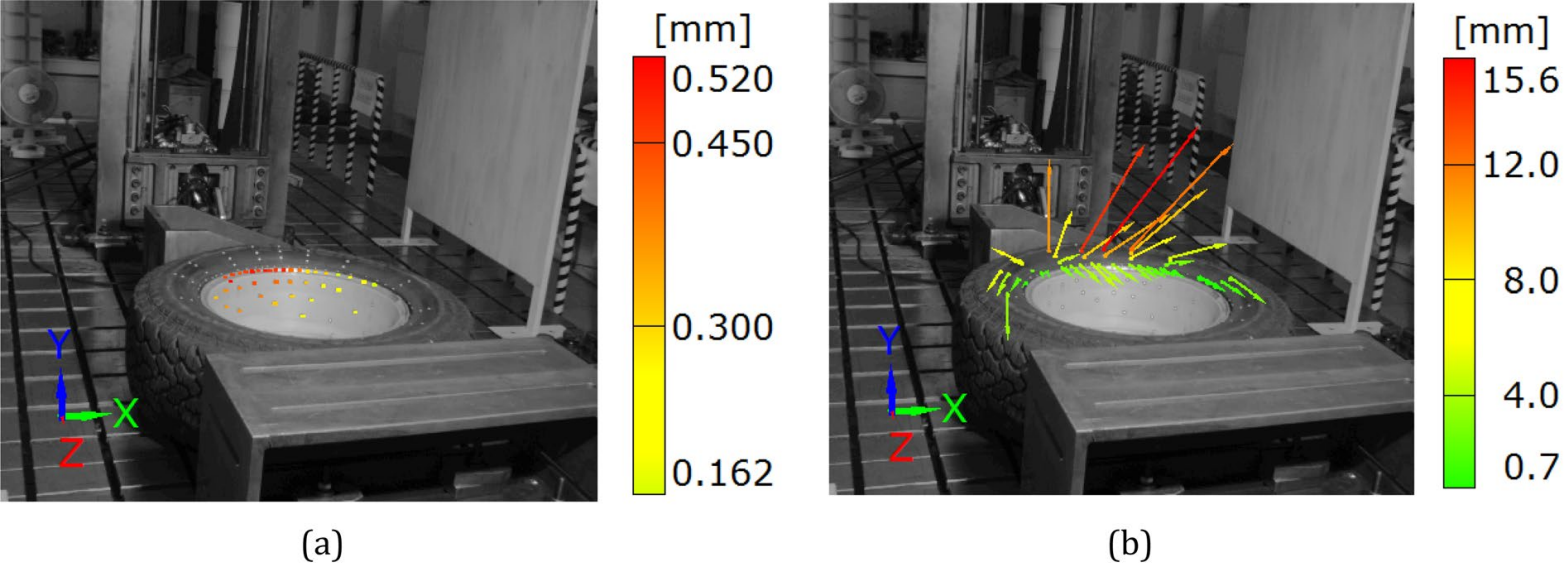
Distributions of the resultant vectors of deflection in the SUV wheel under the force of 11 kN for the rim (a) and tire (b).
